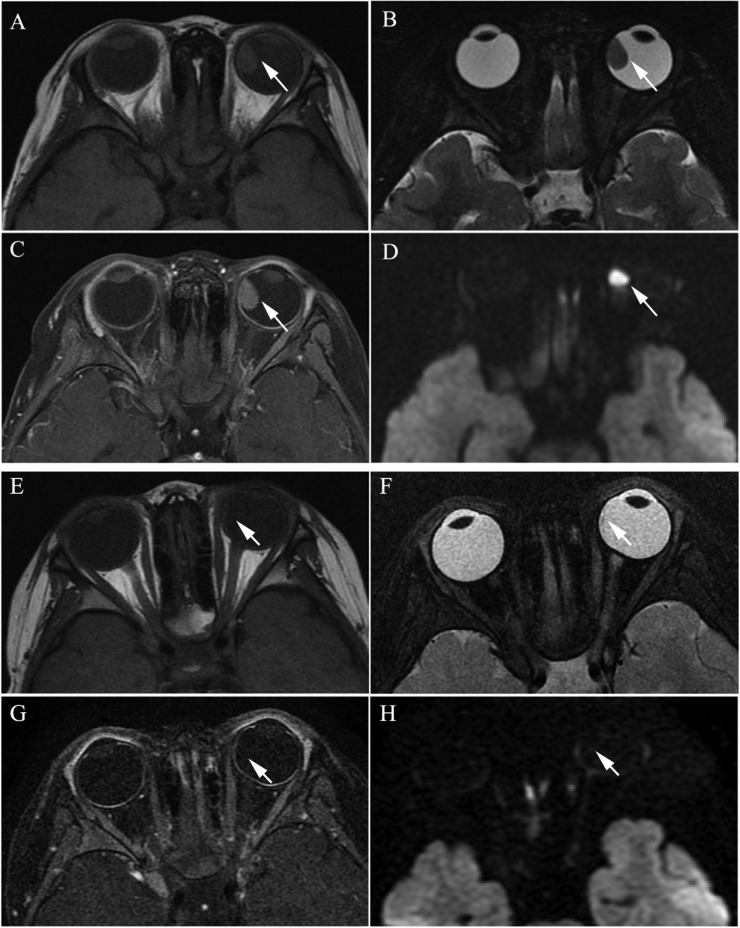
Clinically diagnosed retinoblastoma in a 44-month-old female (patient 2) (A–H) The orbital origin of the tumor was located in the equator of the left globe with the tumor location far from the optic disk, demonstrating a slightly higher signal intensity than the ocular fluid (arrow) in axial T1-weighted MRI (A), low signal intensity (arrow) in the axial T2-weighted MRI (B) and moderate enhancement of the retinoblastoma (arrow) in the axial contrast-enhanced T1-weighted, fat-saturated MRI (C). The axial DWI (D) shows restricted diffusion (arrow) in the tumor. (E–H) A small amount of the residual tumor foci is shown (arrow) in the axial T2-weighted MRI (F) after three cycles of IAC. The axial contrast-enhanced T1-weighted, fat-saturated MRI (G) shows non-enhancement of the residual tumor foci. The axial DWI (H) shows low signal intensity of the residual tumor foci (arrow).
